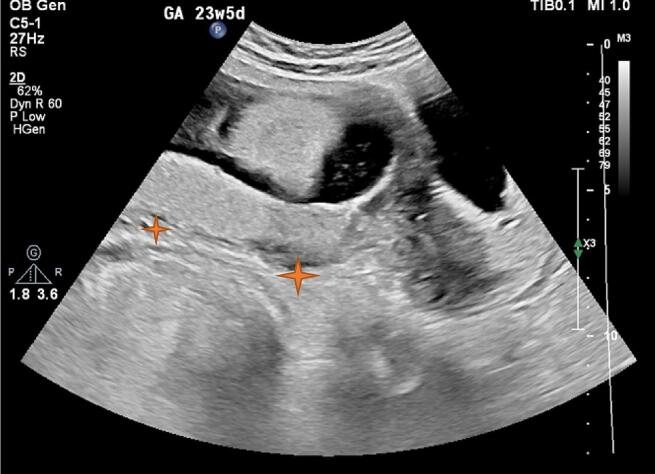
Abdominal sonography: The placenta was seen previa (stars), with no signs of hematoma behind it.
